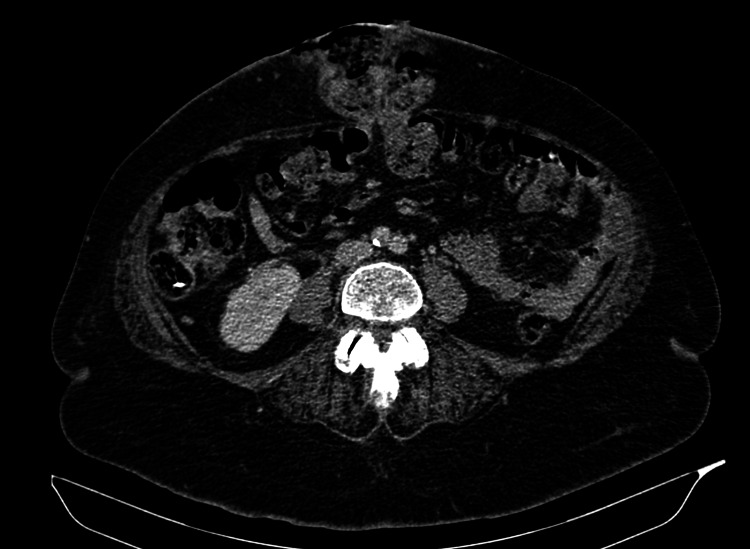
CT of the abdomen showing epigastric hernia containing transverse colon. CT: computed tomography
